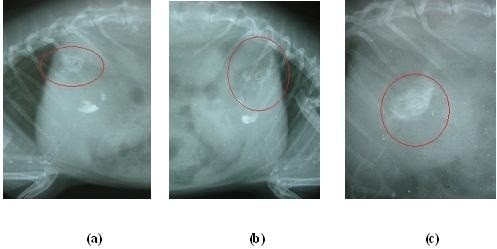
X-ray photographs showing floating ability of F8 formulation at different time interval (a) 6 h, (b) 12 h and (c) 24 h.
